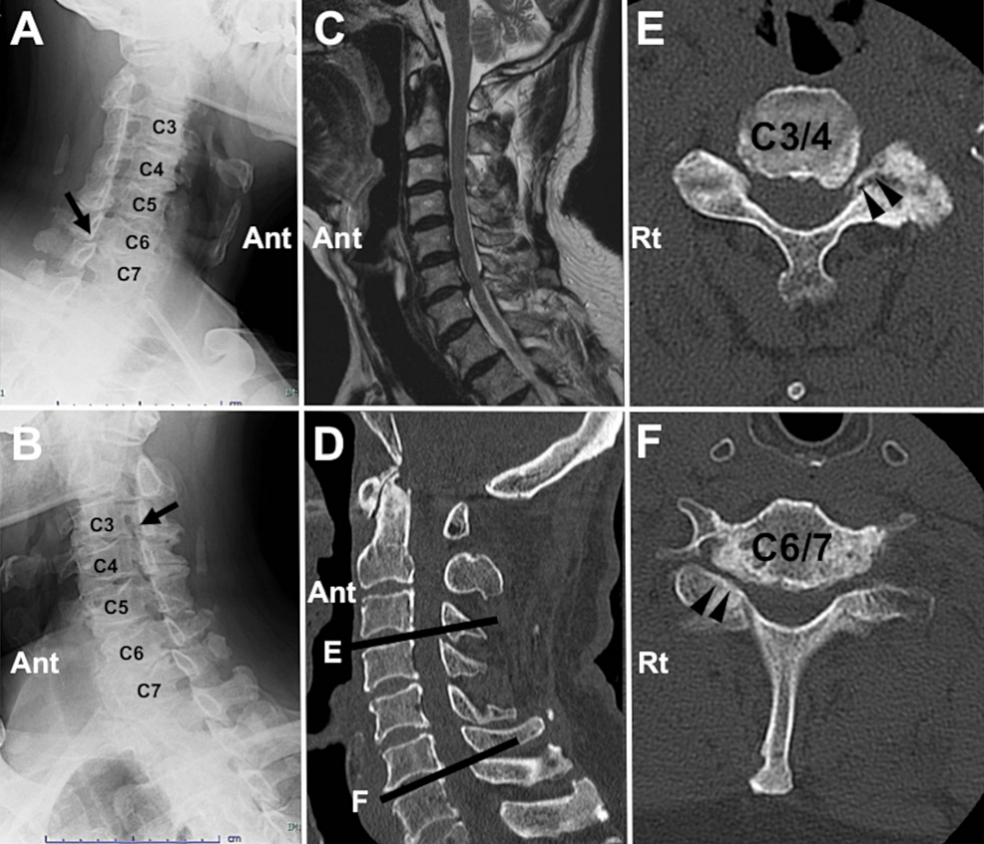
Preoperative radiographic images (Case 1). Oblique X-ray images reveal the stenosis in the right C6/7 foramen (A, black arrow) and in the left C3/4 foramen (B, black arrow). A sagittal magnetic resonance image shows cervical spinal canal stenosis (C). (D-E) Computed tomography images disclose osteophytes forming the foraminal stenosis in the left C3/4 (E) and right C6/7 (F) levels (Ant: anterior, Rt: right).
